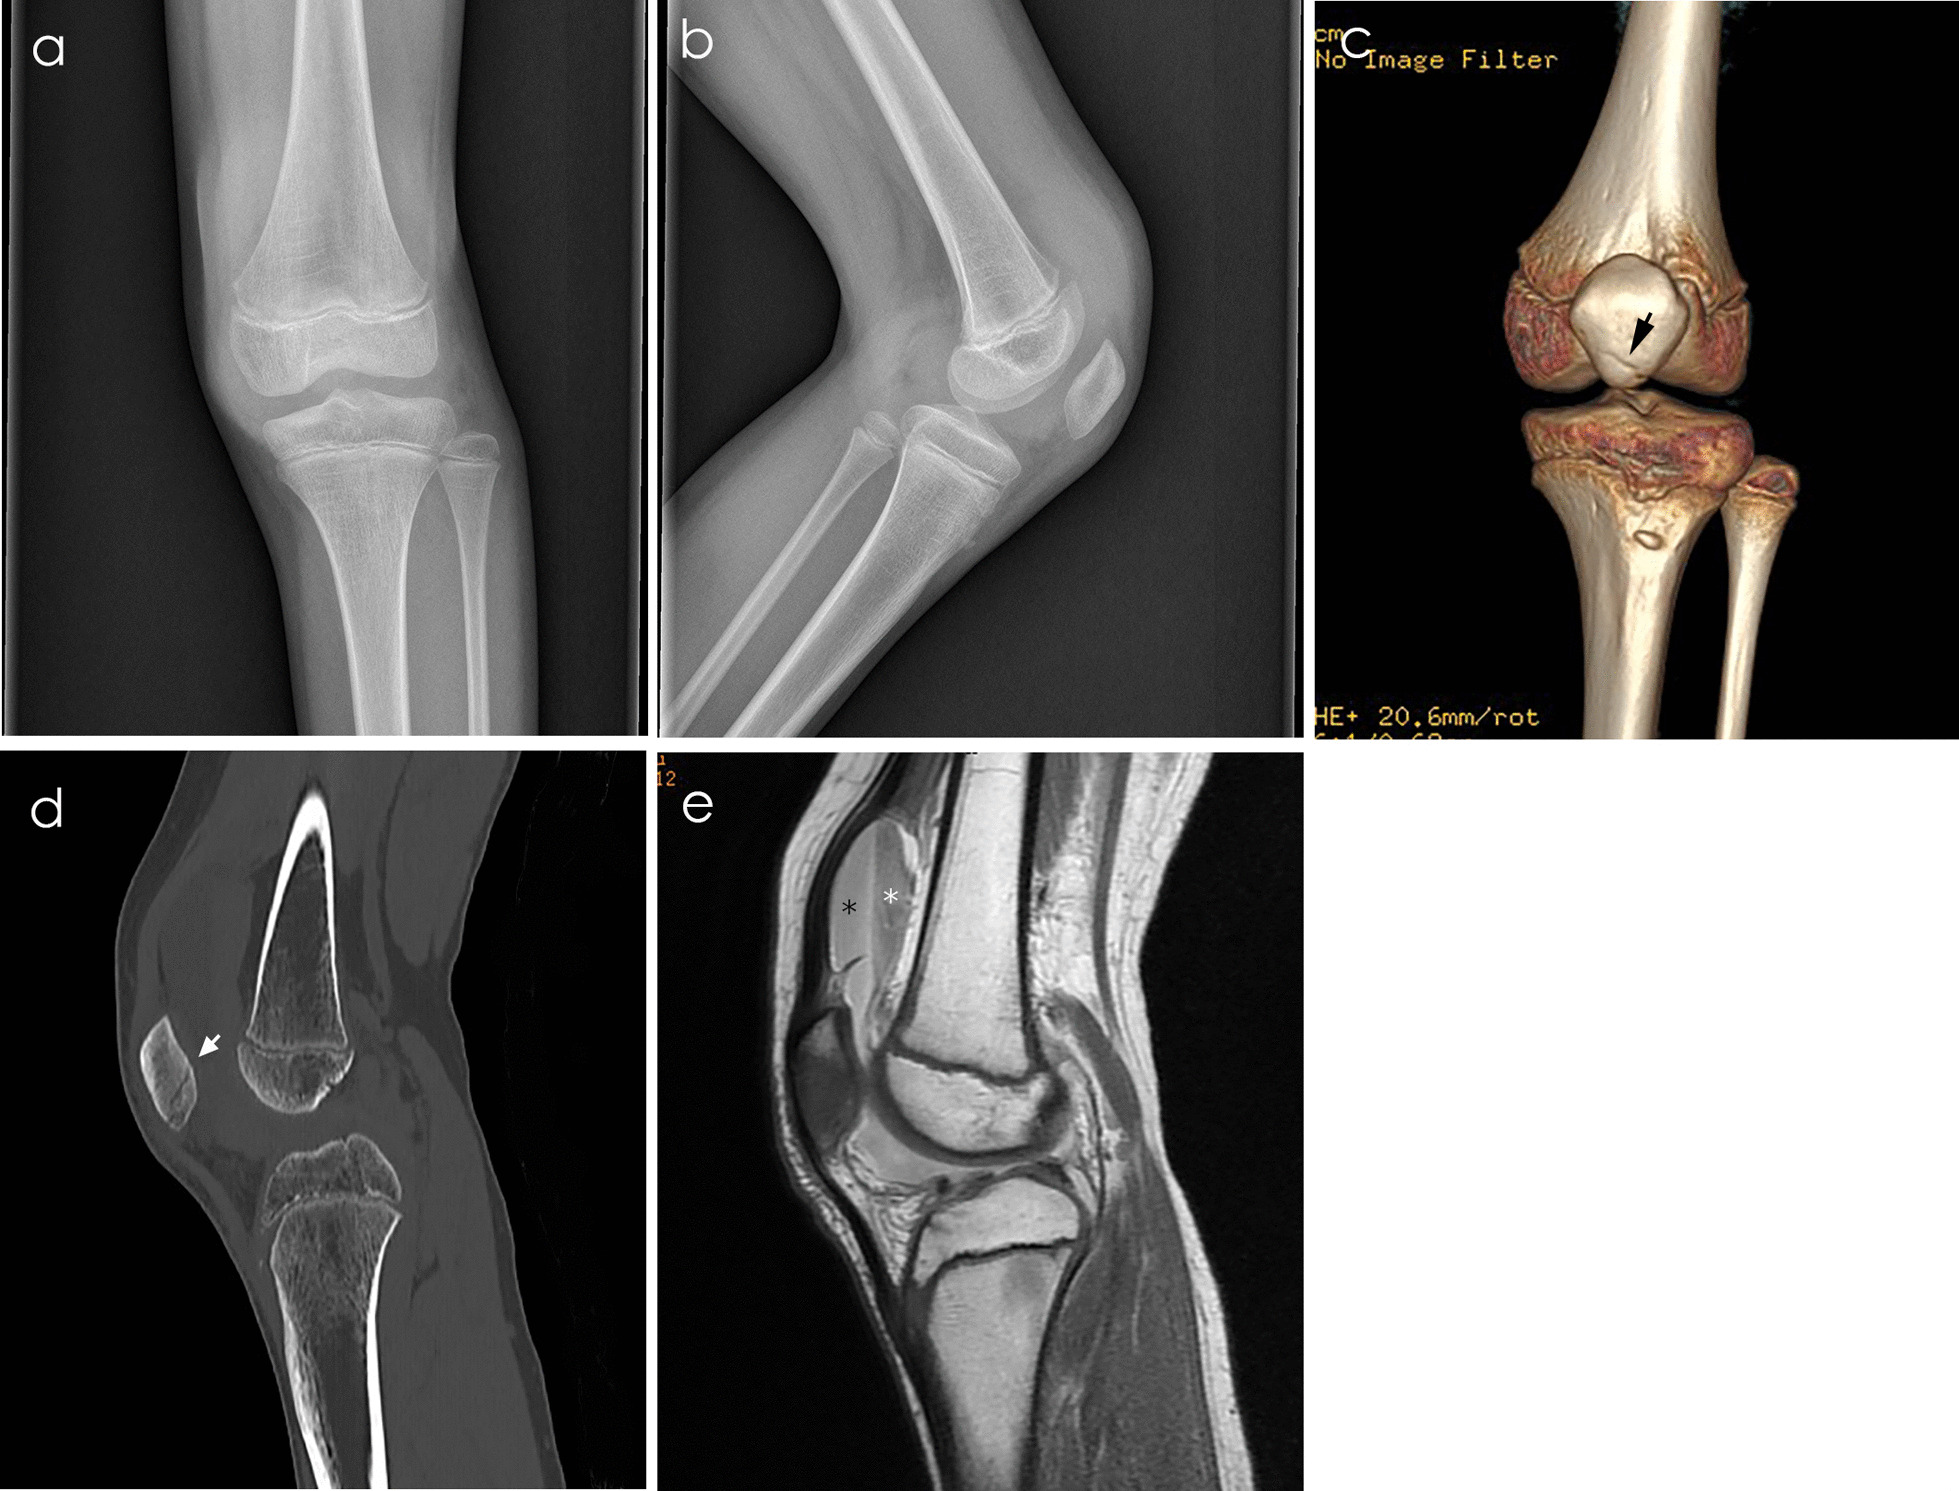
Plain radiograph, CT, and MRI of a 12-year-old boy with right traumatic patellar dislocation. a, b Anteroposterior and lateral knee radiograph did not show the patella fracture line. c CT after reconstruction showed the fracture line at the inferior pole of the patella (black arrowhead). d Sagittal view of CT showing patellar line running through the anterior and posterior edges of the patella (white arrowhead). e SP pouch lipohemarthrosis in T1-weighted MRI. Images show a superior band of fat (black star) and dependent band of red blood cells (white star)
